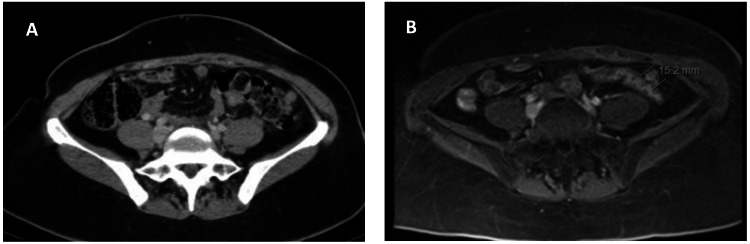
Preoperative CT (A) and MRI (B) scans revealing metastatic peritoneal nodules adjacent to the left colon
